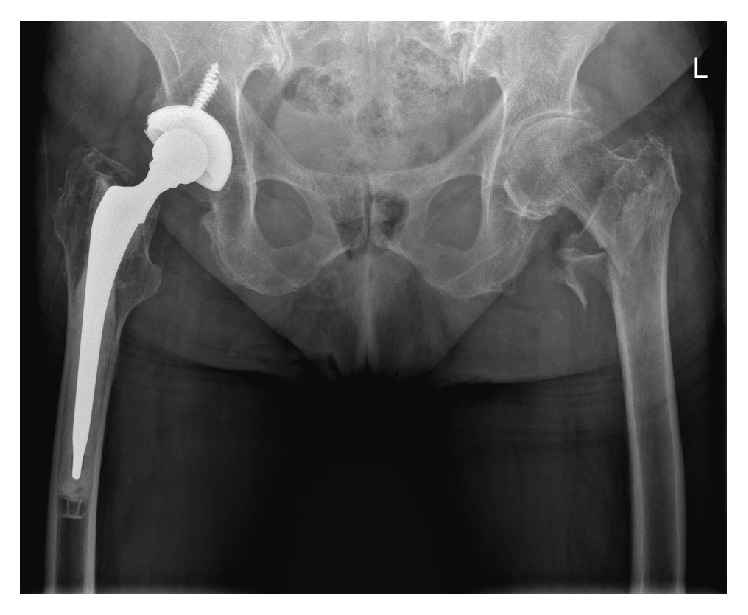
Anteroposterior X-ray showing a pertrochanteric fracture of the left femur.
